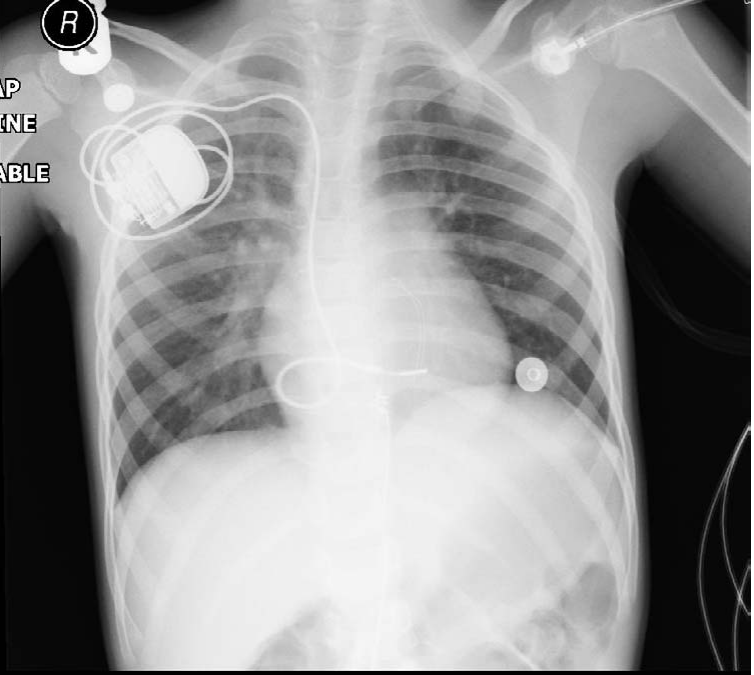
X-ray depicting the endocardial pacemaker inserted through the right subclavian vein.
